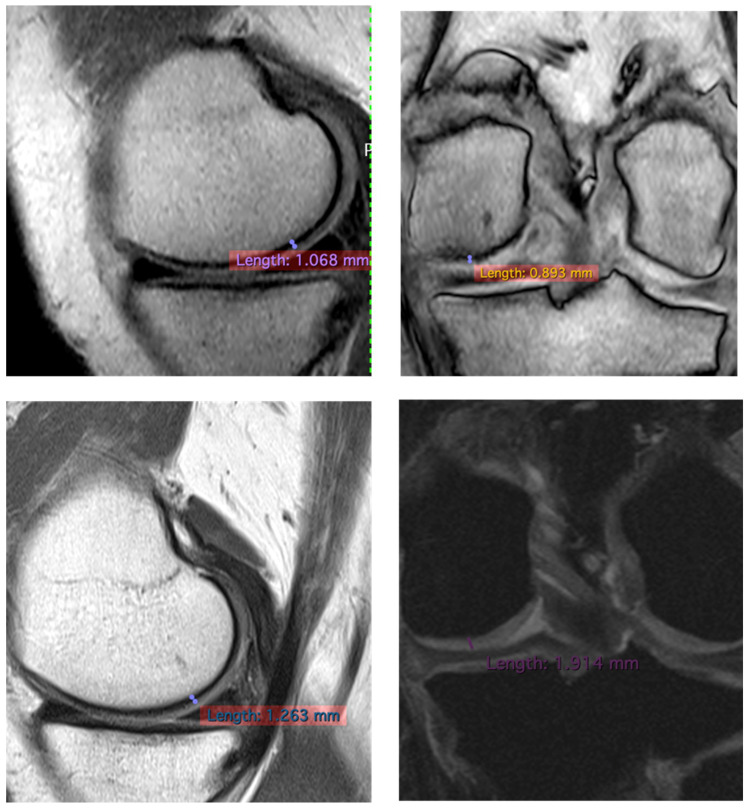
Representative images of MRI of patient no 2 at 3 years. Images of femoral lateral condyles and measurement of thickness cartilage. We state that different MRI machines and different sequences were used for each patient.
